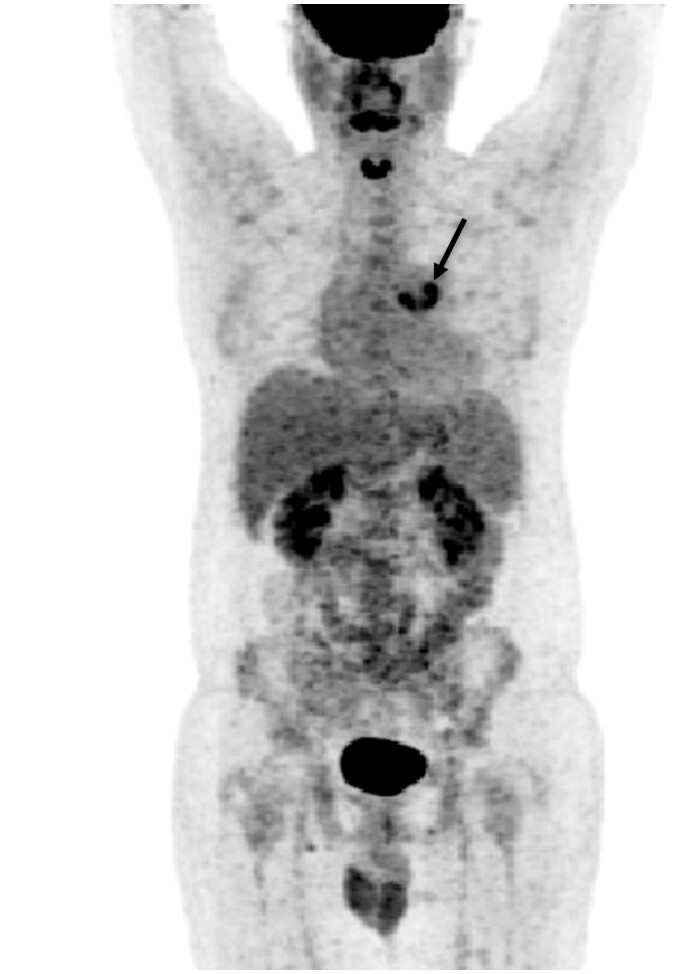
MIP image from 18FDG PET/CT scan showing intense focal uptake in the prosthetic valve (black arrow) in keeping with infective endocarditis.
